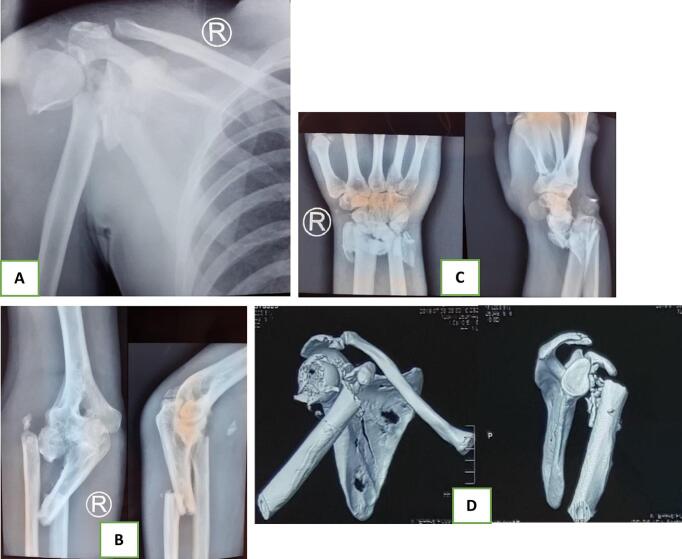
Radiographic series showing multiple fractures and dislocations of the right upper extremity; (A) shoulder anteroposterior, (B) elbow anteroposterior and lateral, (C) wrist anteroposterior and lateral, and (D) shoulder CT scan.
